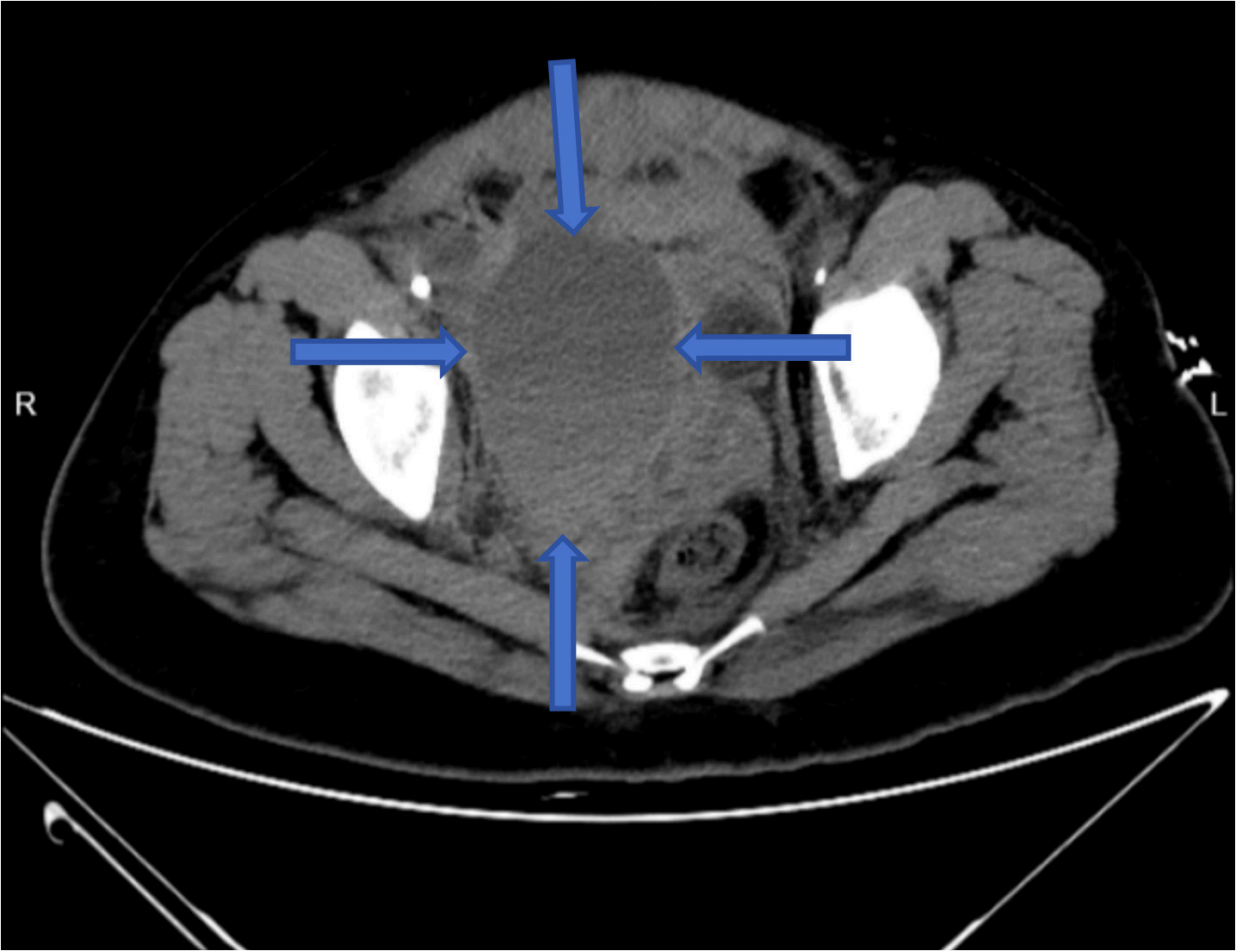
Axial abdominal CT scan showing a hematoma (indicated by arrow).
